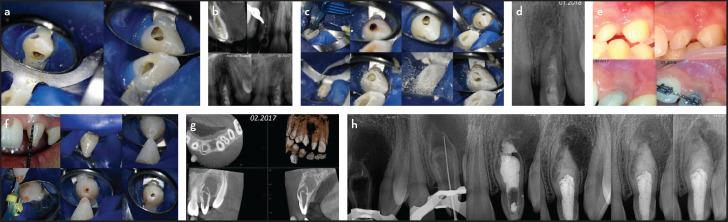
Type II DI cases: Case 3 depicts Type IIC root canal configuration (a) and its management using revascularization technic (b) followed by luting of glass fiber post (c) with 1 year follow up radiograph (d) and comparative preop and postop follow up photographs (e) Case 4 depicts Type IIC root canal configuration (f, g) and its management via placement of collagen and biodentine with post op and 3 years follow up radiograph (h)
